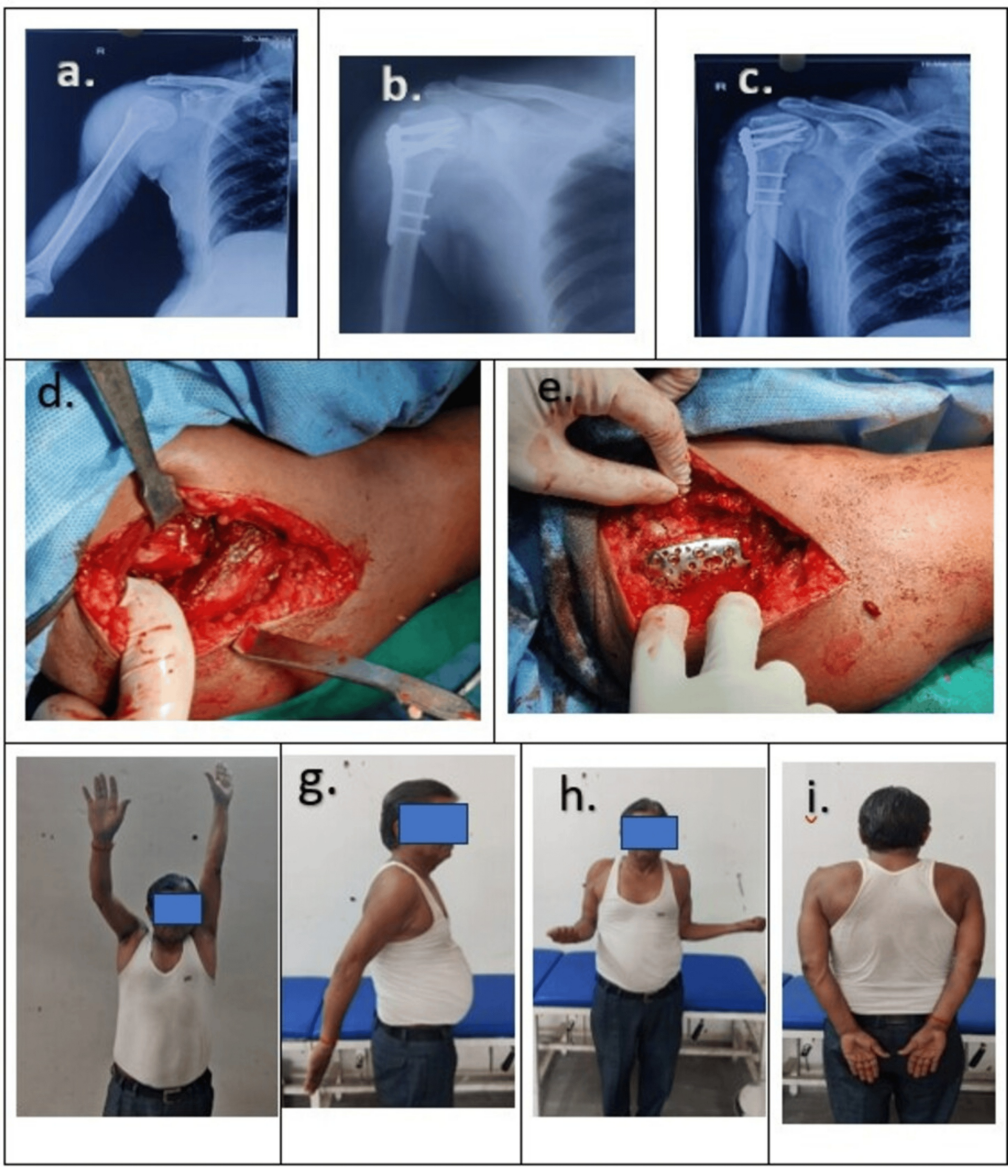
Patient with PHF treated by PHILOS plate (a) Preoperative X-ray of the right shoulder joint; (b) postoperative X-ray; (c) six months follow-up X-ray; (d) opening of fracture by deltopectoral approach; (e) final fixation of the PHILOS plate; (f, g, h, i) functional outcome PHF: Proximal Humerus Fractures; PHILOS: Proximal Humerus Internal Locking System
